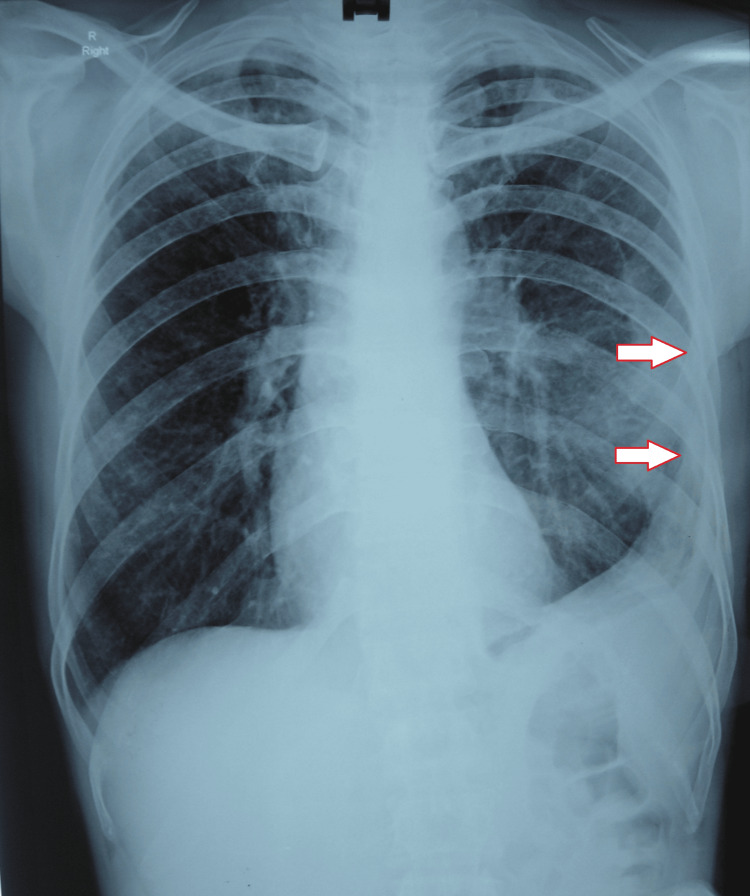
Postoperative chest X-ray showing left pleural thickening with no pleural collection.
